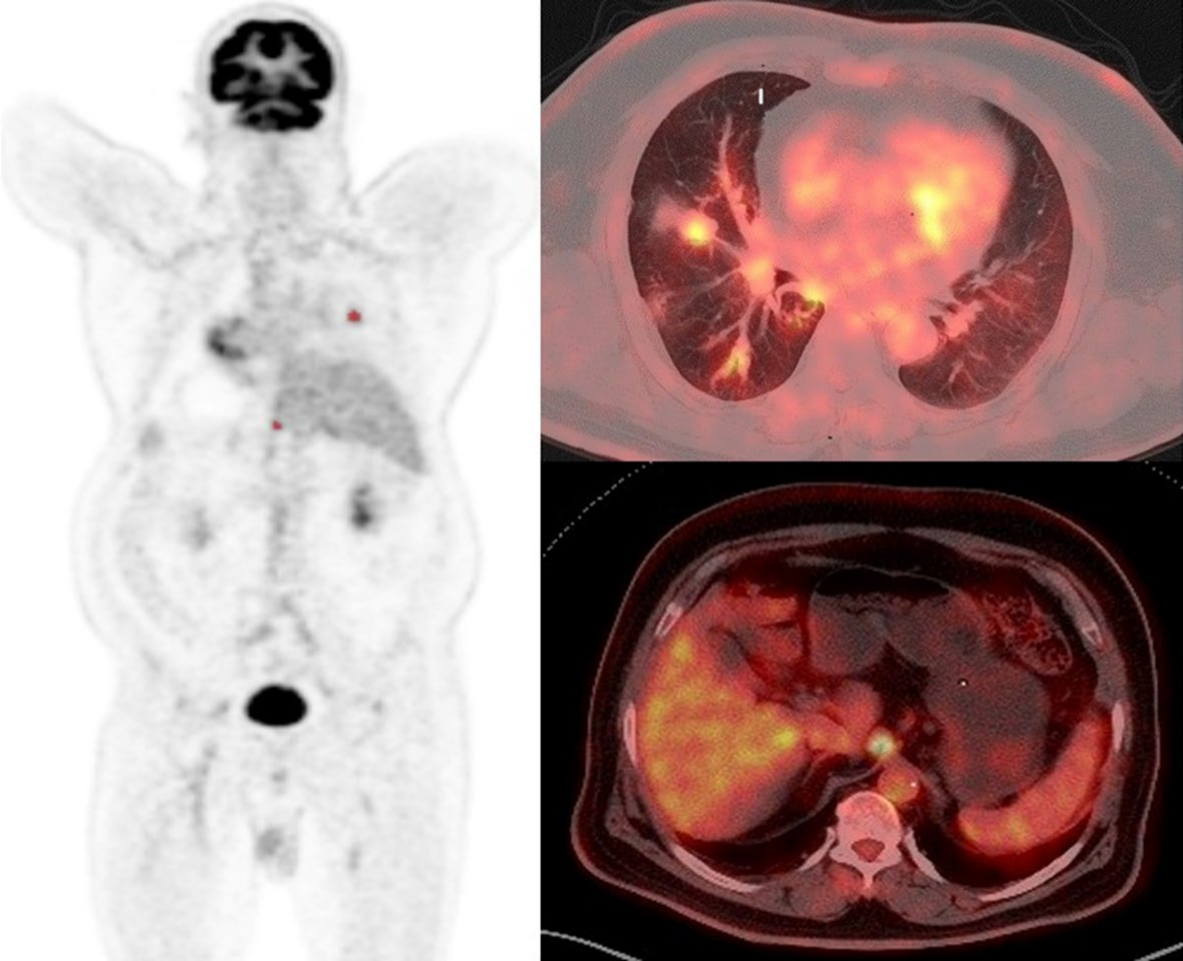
Representative ^18^F-FDG PET-CT MIP (maximum intensity projection) and axial images of a patient with low whole-body tumor burden (WBTB). A 62-year-old male patient with pathologically proven right middle lobe NSCLC, adenocarcinoma. FDG uptake was increased abnormally corresponding to the right lung primary lesion, another focal FDG uptake is noted at abdominal LN. Corresponding SUVmax, SUVpeak, SUVmean, tu MTV, tu TLG, WB MTV, and WB TLG were 3.48, 2.71, 2.04, 5.23, 10.56, 30.8, 56
